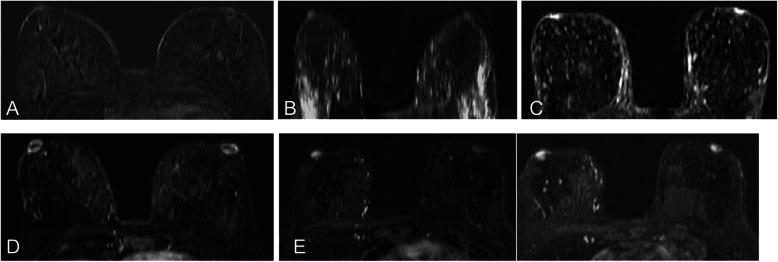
Physiological enhancement in the nipple-areolar complex. Axial contrast-enhanced T1-weighted spoiled gradient-echo (subtracted) images show various degrees of enhancement in a normal nipple, including none (a), mild symmetric enhancement (b), intense symmetric enhancement (c), a thin symmetric ring of enhancement (d), and asymmetric early enhancement with symmetric late enhancement (e)
